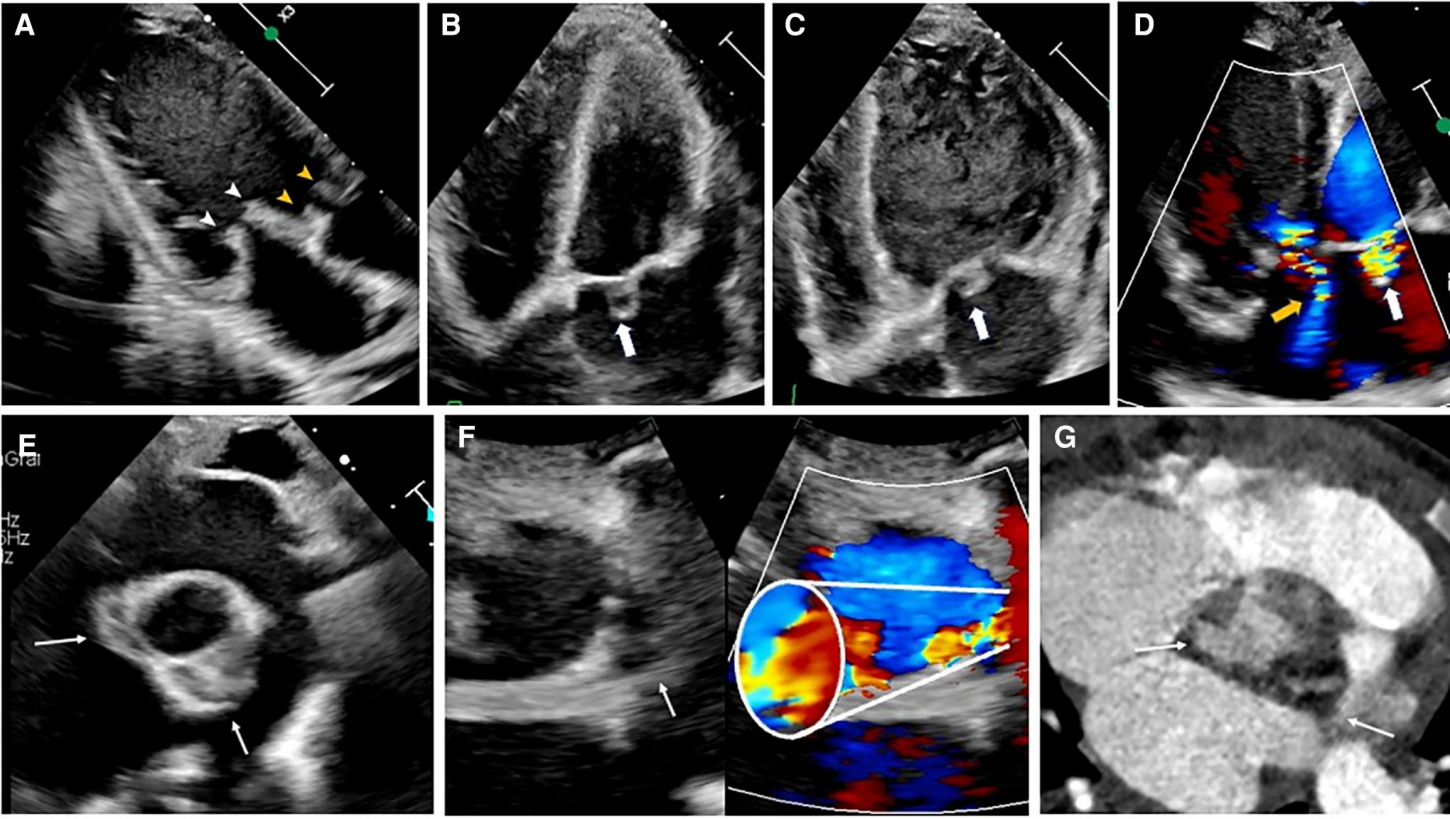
(*A–F*) Transthoracic echocardiography. (*A*) Three-chamber view that shows multiple mobile vegetations in mitral (*white arrow heads*) and aortic (*yellow arrow heads*). (*B–D*) Four-chamber view revealing a mitral pseudoaneurysm (*white thick arrow*) with systolic expansion (*B*), diastolic collapse (*C*), and pulsatile flow (*D*); tricuspid regurgitation is also observed (*yellow arrow in D*). (*E–F*) Paraesternal short axis at the aortic level demonstrating two aortic pseudoaneurysms (*white thin arrows*); zoomed dual image with colour Doppler showing bidirectional flow (*F)*. (*G*) Cardiac angio-tomography depicting both aortic pseudoaneurysms.
